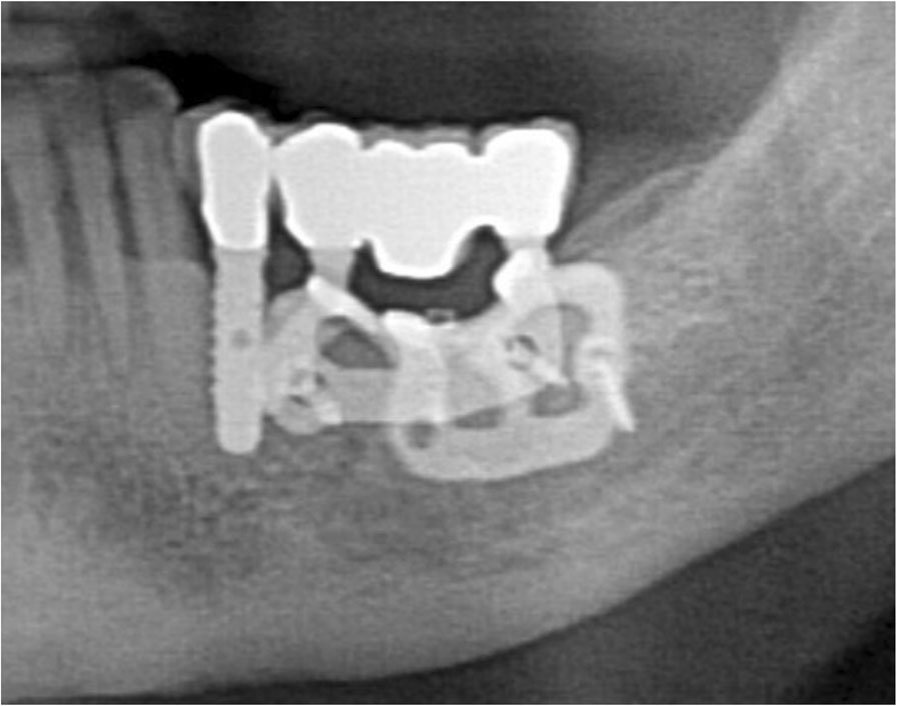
The zirconia-ceramic restoration at the 1-year follow-up control, radiographic control
